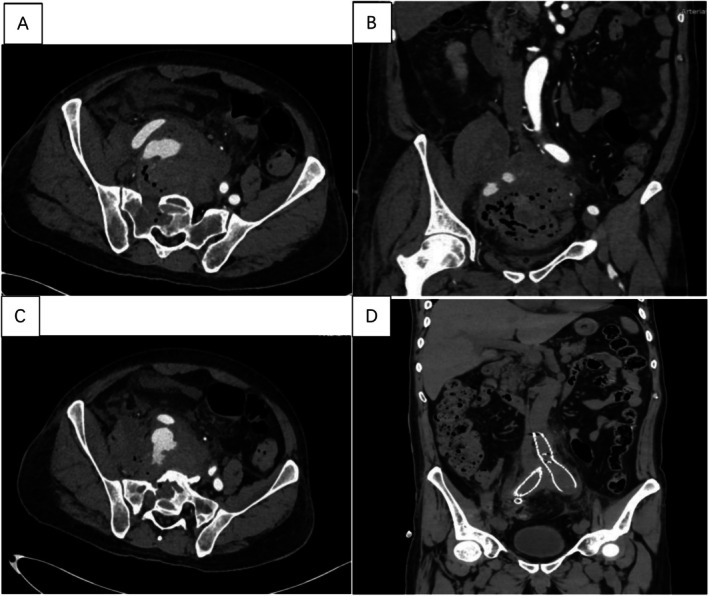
From onset to postoperative abdominal CT imaging findings. (A) Abdominal enhanced CT: Infectious involvement of the sacrum. (B) Abdominal enhanced CT: Abscess formation anterior to the sacrum and within the right lumbar region. (C) Abdominal enhanced CT: Ruptured pseudoaneurysm of the right common iliac artery. (D) Abdominal CT plain scan: Postoperative imaging showing stent graft placement in the infrarenal abdominal aorta and bilateral common iliac arteries.
